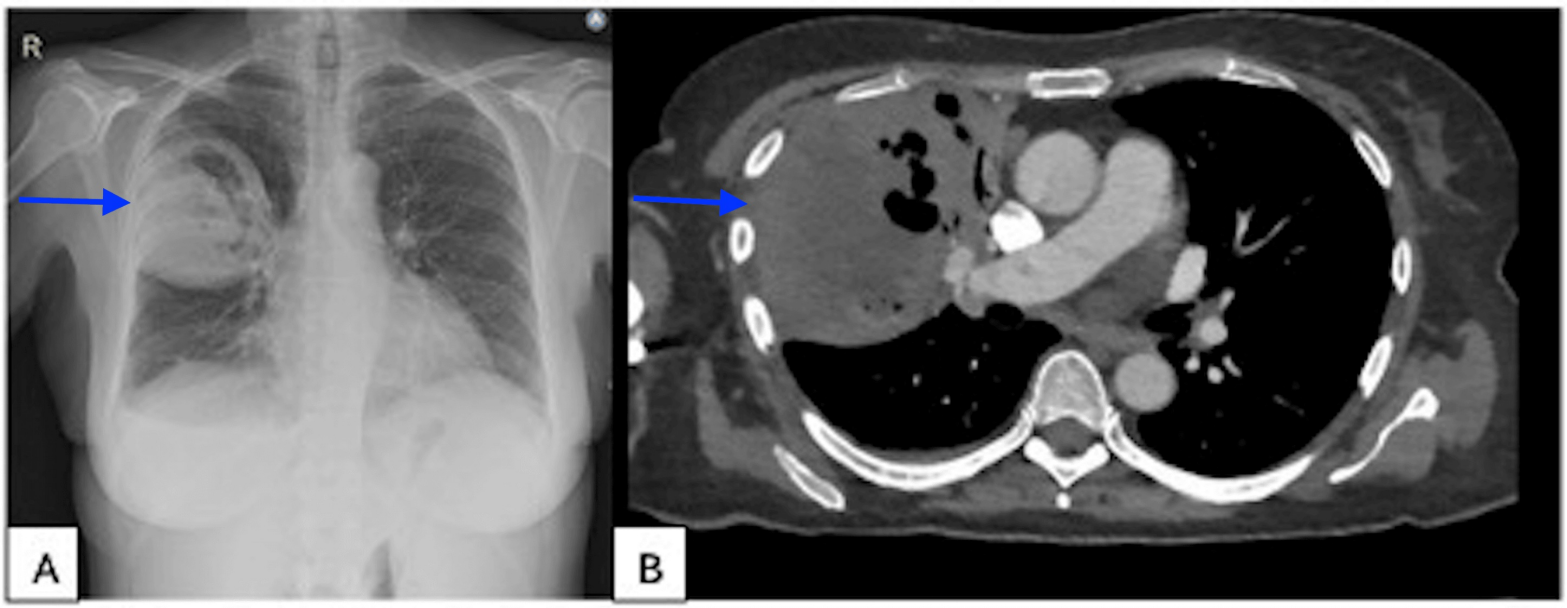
(A) X-ray after initial treatment shows cavitation of the initial consolidation four weeks after start treatment. (B) CT scan of the thorax confirms these finding more in detail: a thick-walled cavity with a diameter of 9 cm and air-fluid levels CT: computed tomography
